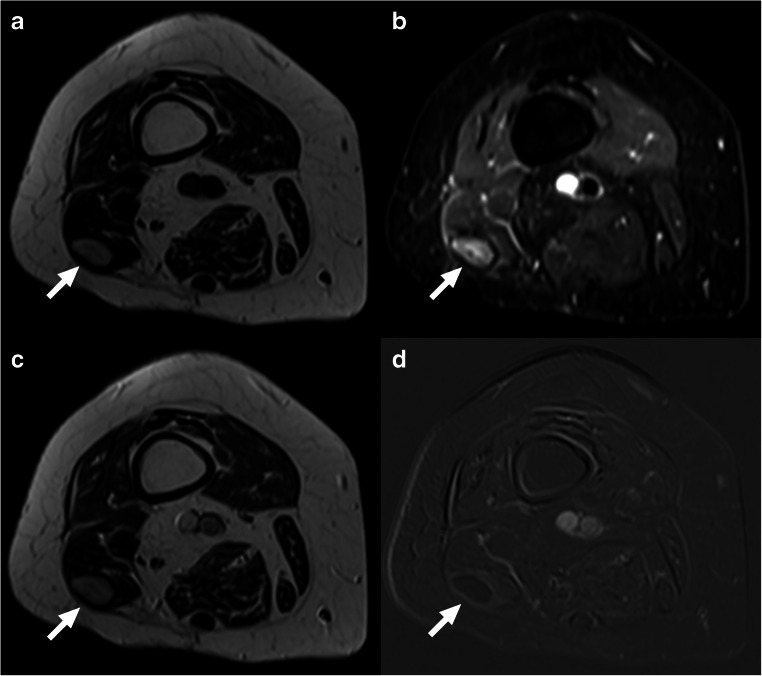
Example of a subacute hematoma with high T1 and T2 signal intensity (not to be confused with residual tumor) in a 77-year-old woman with pleomorphic sarcoma who had undergone a Whoops procedure at the lateral side of the distal right upper leg. Axial T1-weighted (a), fat-suppressed T2-weighted (b), gadolinium chelate-enhanced T1-weighted (c), and subtraction (a subtracted from c) gadolinium chelate-enhanced (d) images at the level of the distal right upper leg. There is a lesion with high T1 and T2 signal intensity in the distal biceps femoris muscle (deep-seated location) and a surrounding low signal intensity rim (a and b, arrows). The lesion also has a high signal on the gadolinium chelate-enhanced T1-weighted image (c, arrow), and it remains unclear if the lesion is enhancing. However, the subtraction gadolinium chelate-enhanced image clearly shows no nodular or mass-like enhancement (d, arrow), which favors subacute hematoma and makes residual tumor unlikely. Pathological examination after second surgery showed hematoma and no residual tumor
